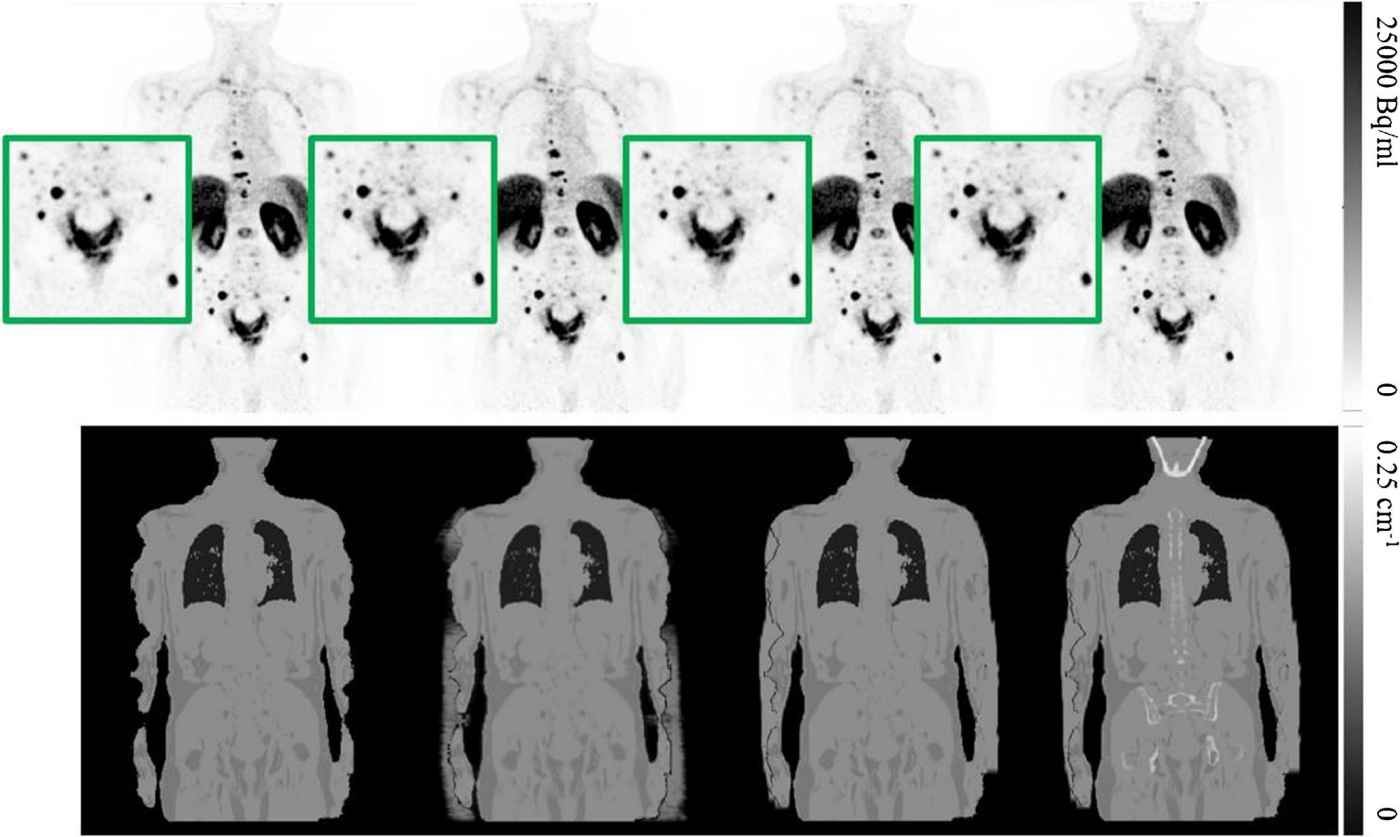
Four reconstructed ^18^F-PSMA PET images (top) with the corresponding four attenuation maps (bottom), left to right: standard Dixon-VIBE, Dixon with MLAA, Dixon with HUGE, and Dixon with HUGE and bones
